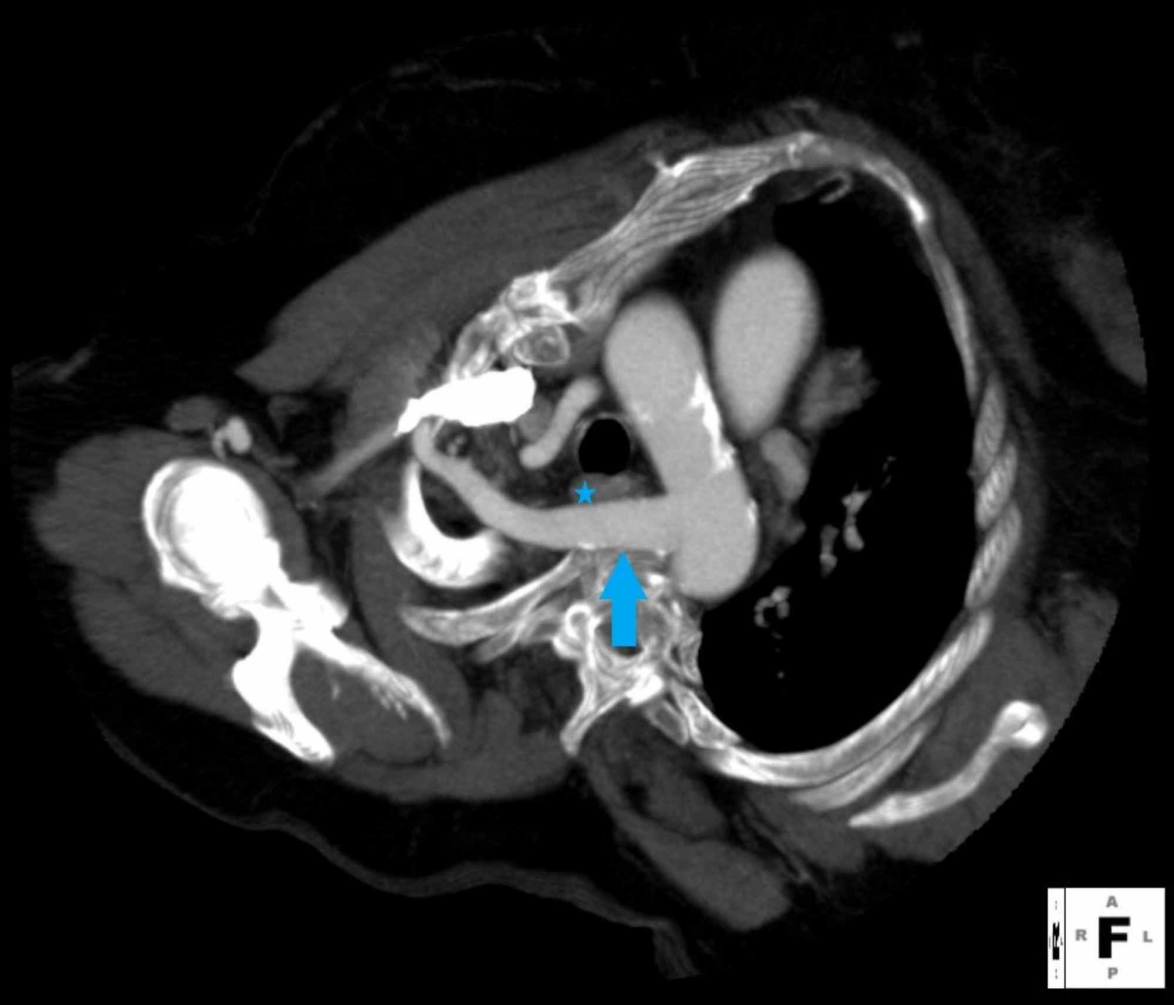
Chest contrast-enhanced reconstructed computed tomography (CT) in the venous phase, axial view, showing an aberrant right subclavian artery (arrow), which passes behind the esophagus (asterisk) and trachea.
